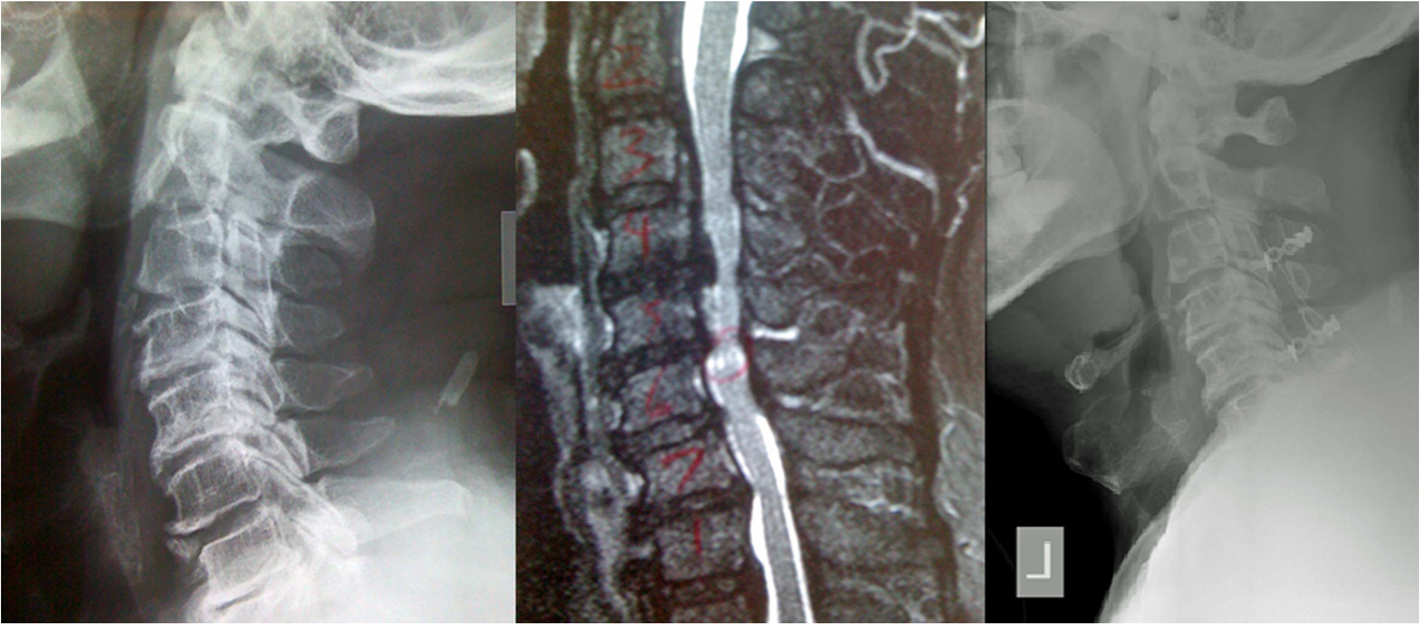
Pre-operative lateral x-ray of a patient with multi-level ossification of the posterior longitudinal ligament (left) and significant narrowing of spinal canal with myelomalacia at C5-C6 as seen on the T2-weighted MRI (middle). A laminoplasty fixed with miniplates was performed (right)
